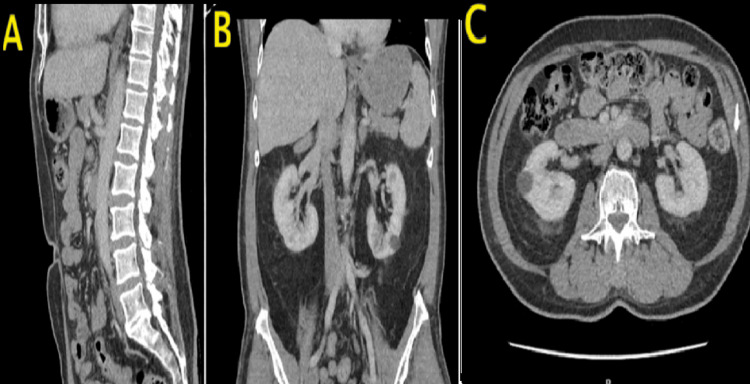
CTAP showing no evidence of malignancy Sagittal (A), coronal (B), and axial (C) views of the abdomen and pelvis showing no evidence of malignancy CTAP: CT scan of the abdomen and pelvis
